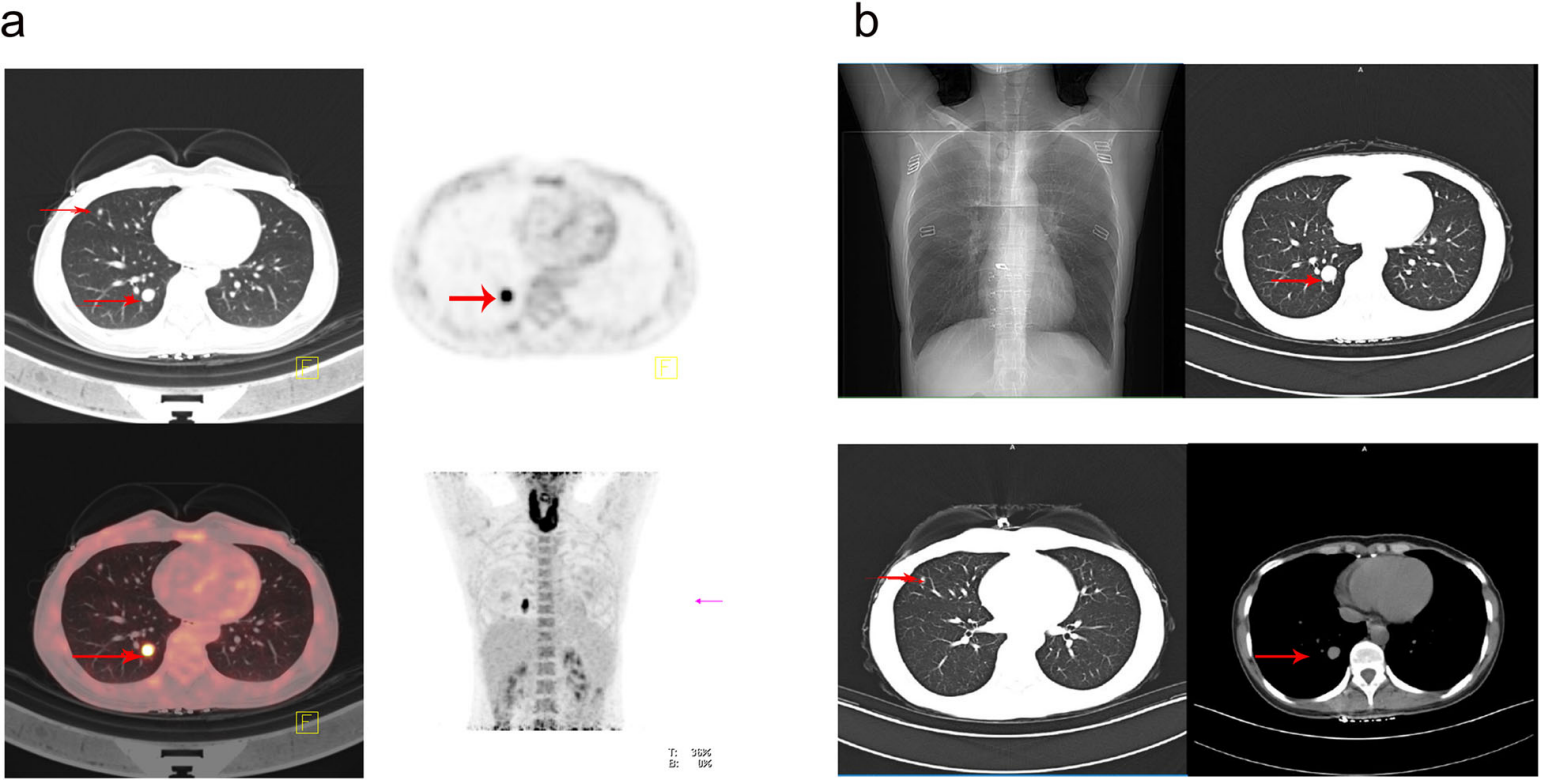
a PET-CT revealed multiple nodules in the right middle lobe of lung and a nodule with abnormal metabolism increase in the right lower lobe; b Chest CT showed the tumor’s size in the right lower lobe was increased
